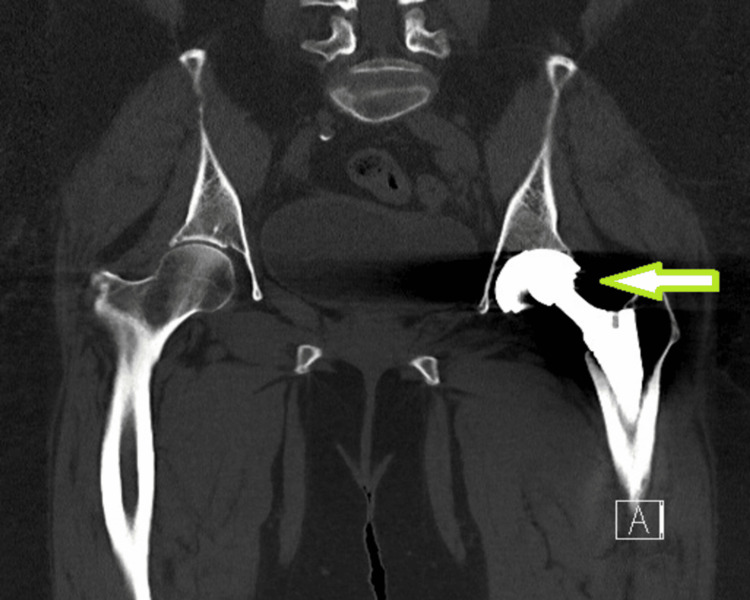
Pre-operative computed tomography scan coronal section of pelvis with both hip joints. Green arrow pointing at the eccentric and lateral position of left femoral head component within the acetabular shell.
